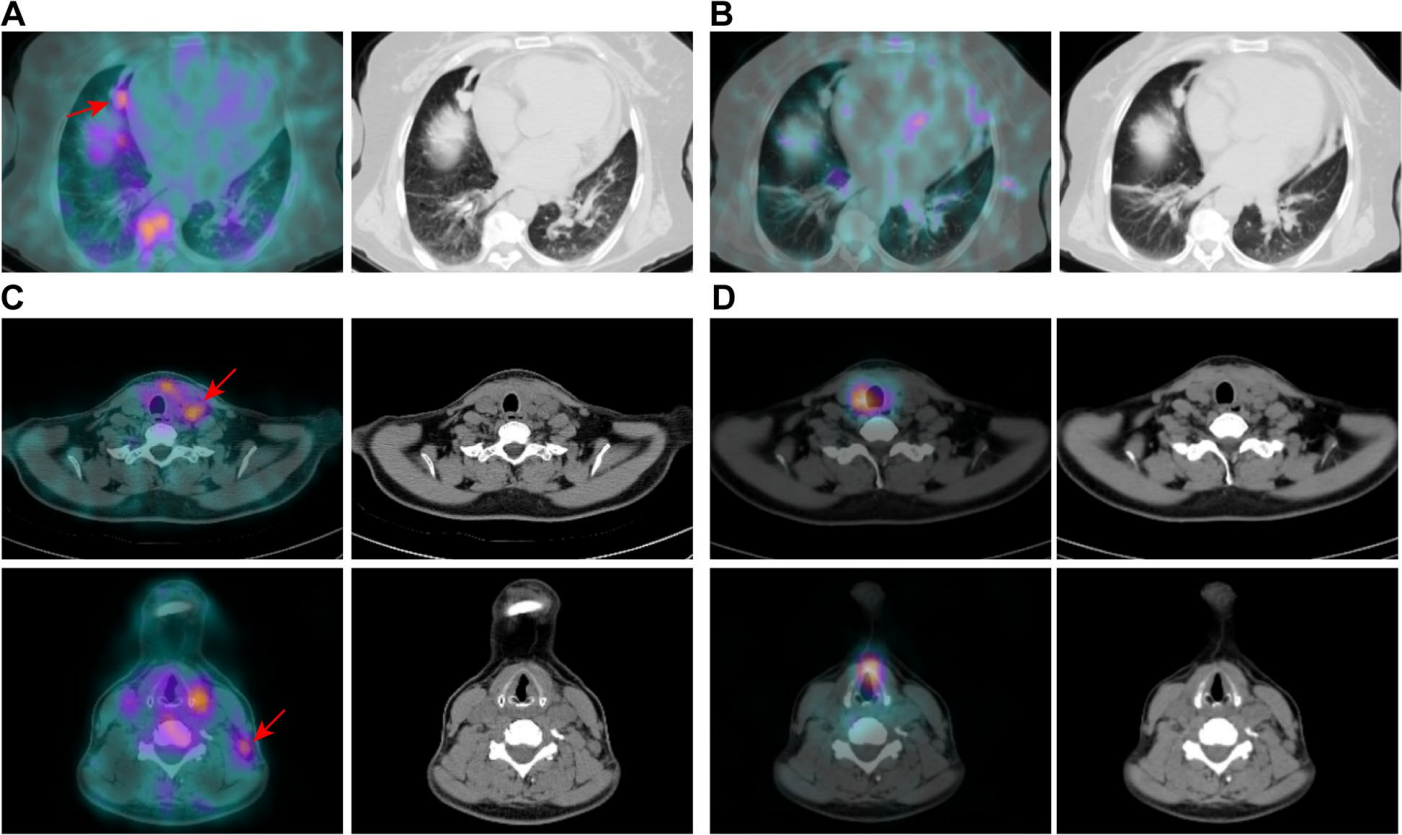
Representative ^99m^Tc-3PRGD_2_ SPECT/CT images of patients with ^131^I negative and ^99m^Tc-3PRGD_2_ positive lesions. A
